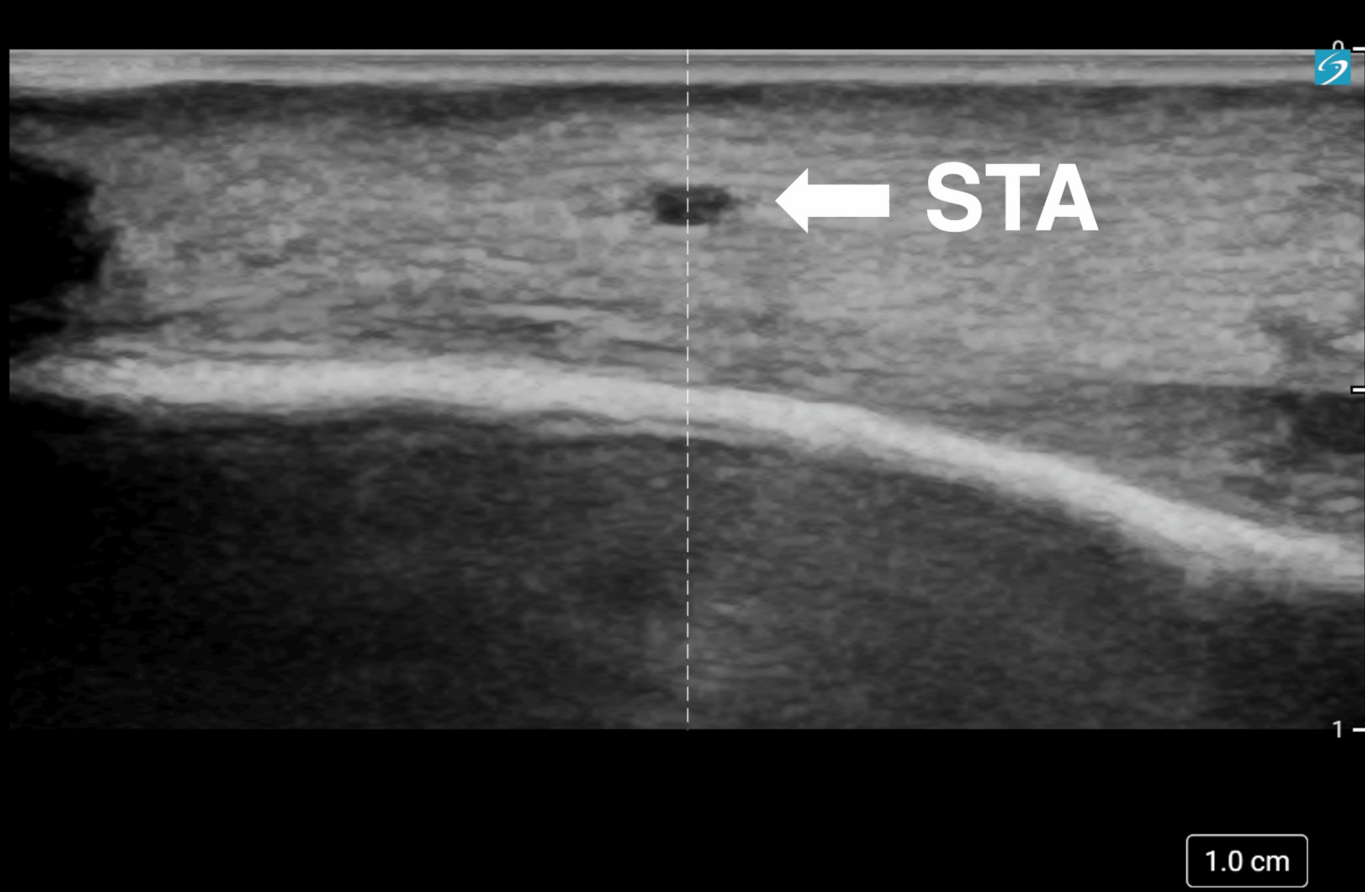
Preoperative ultrasound image of the left STA Short-axis high-frequency ultrasound image of the left STA at a depth of approximately 2 mm from the skin surface. The inner lumen measured 0.47×0.35 mm on preoperative assessment. STA: superficial temporal artery
